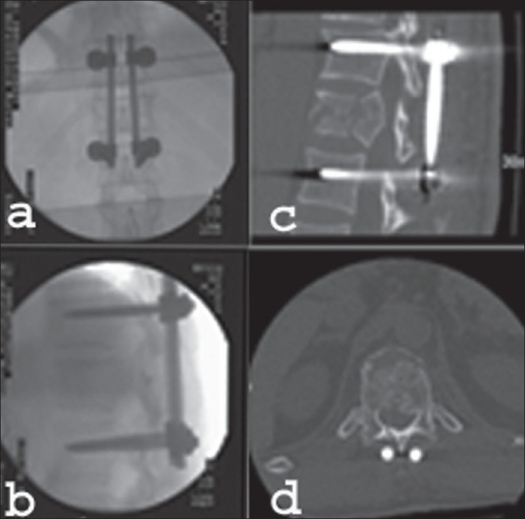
Plain x-ray AP (a) lateral (b) sagittal reconstruction CT scan (c) and axial CT scan (d) shows dorsal reduction and instrumentation of T12-L2 using internal fixator
